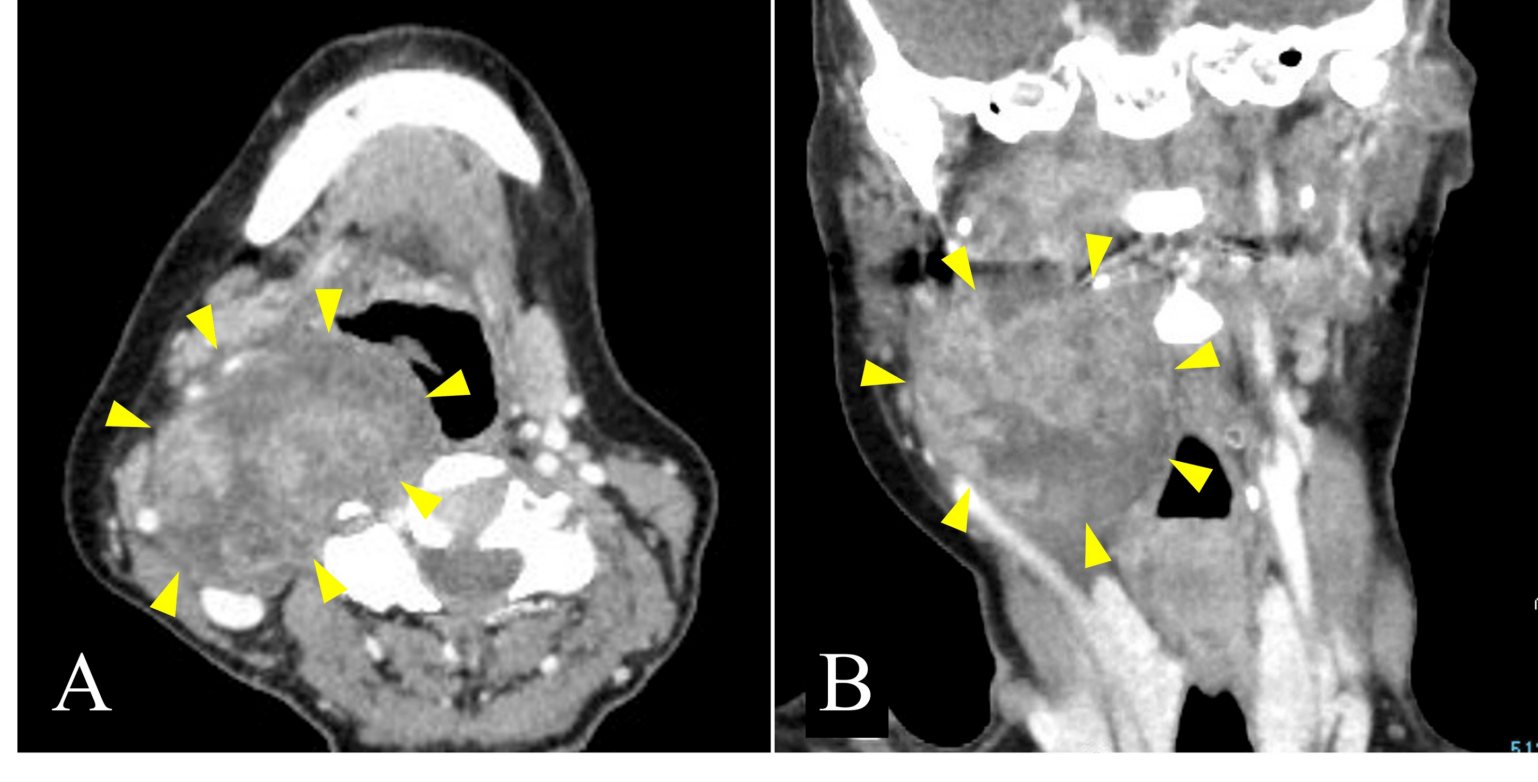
Contrast-enhanced CT image: (A) axial section; (B) coronal section. A mass with a maximum transverse diameter of 7 cm is seen in the right parapharyngeal space. The tumor is heterogeneously contrasted. The internal carotid artery and internal jugular vein are compressed laterally.
